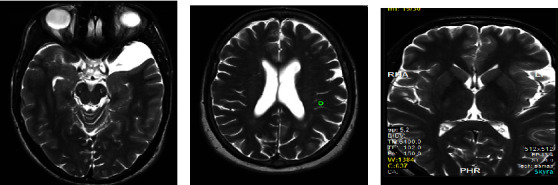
The type of lesion presented in MR images. (a) Axial T2W lesion was seen in the left temporal region; mostly, it is an arachnid cyst. (b) Axial T2 WI shows a prominent ventricular system and edema. (c) Axial T1 and T2 in the left parietal region suggestive of old stroke.
